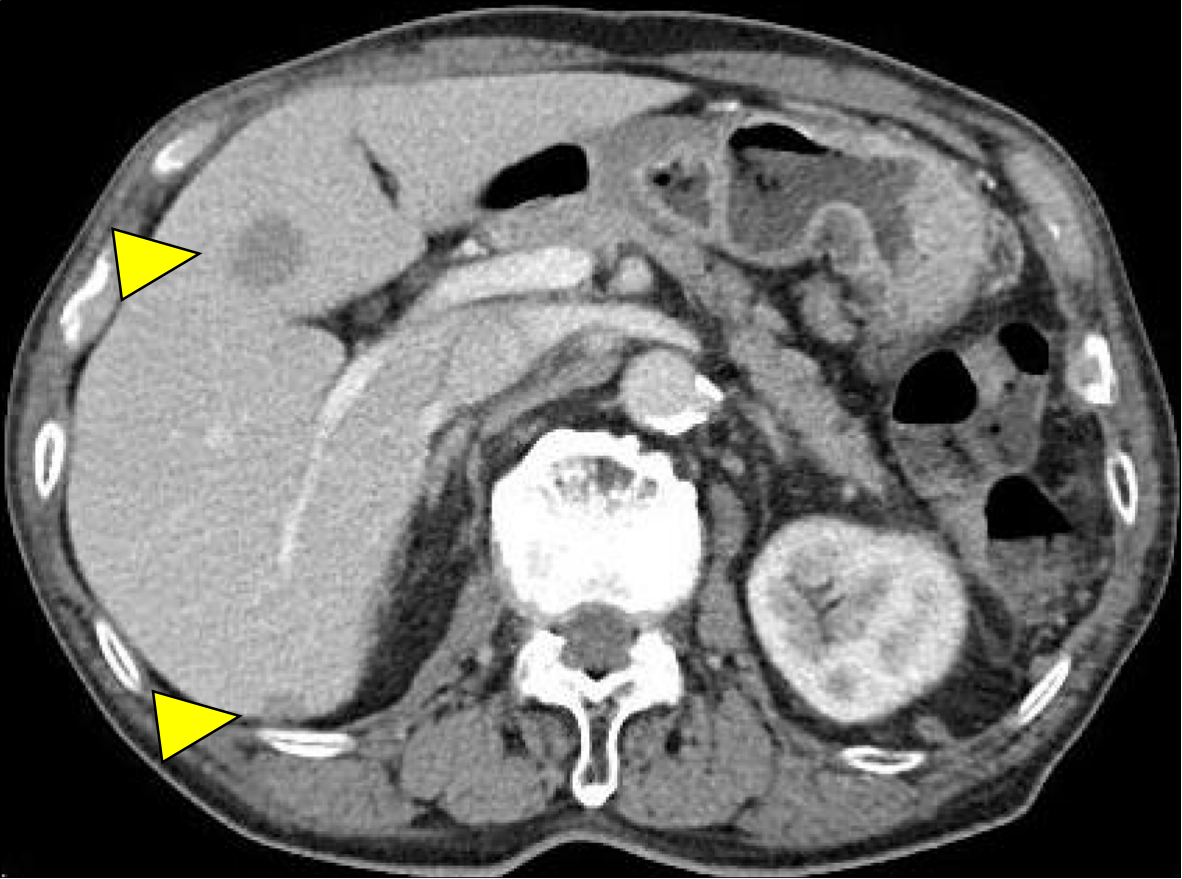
Abdominal computed tomography scan 6 months after surgery revealed multiple metastatic lesions in the liver. A total of 5 lesions were found
